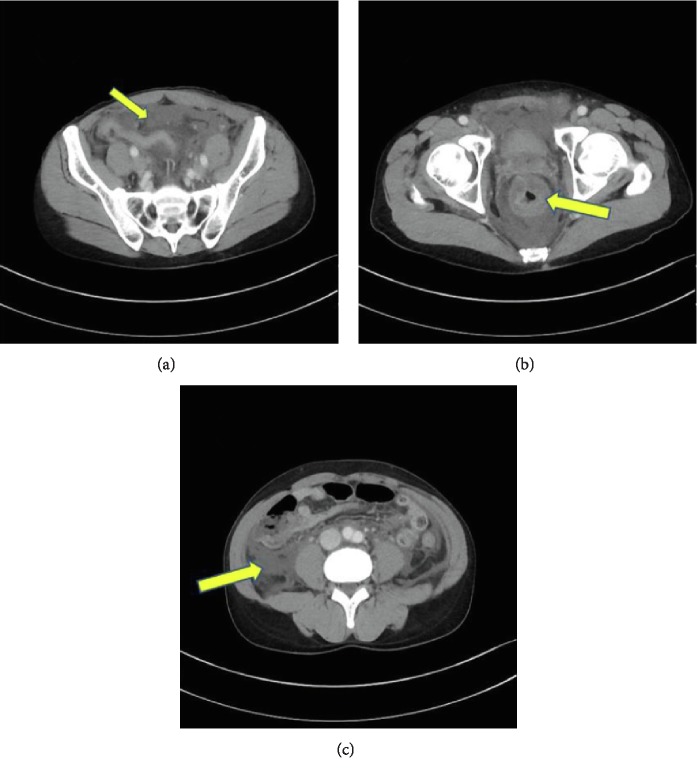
Abdominal computed tomography findings in a patient with primary peritonitis. (a) Computed tomography indicated a small amount of ascites. (b) Computed tomography showed edema of the intestinal membrane. (c) Computed tomography showed edema of the retroperitoneum.
